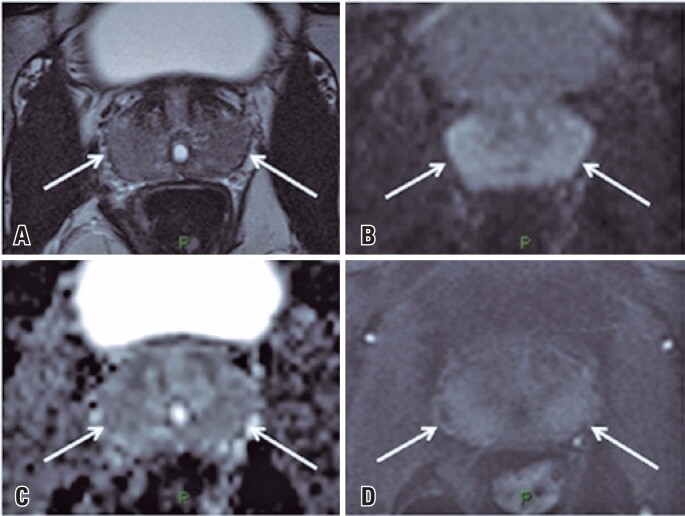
Prostate magnetic resonance imaging of a 31-year-old asymptomatic man to evaluate an epididymal cyst. The exam shows diffuse hyposintensity on T2W (arrows in A), with areas of diffusion restriction seen on DWI and ADC map (arrows in B and C) and early diffuse contrast enhancement (arrows in D)
